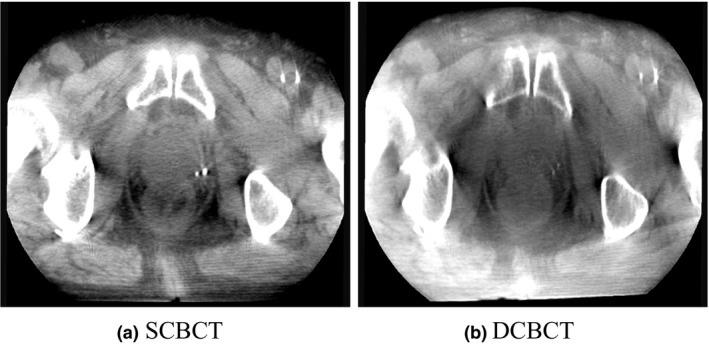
Axial images of the pelvis region obtained using (a) SCBCT and (b) DCBCT.
